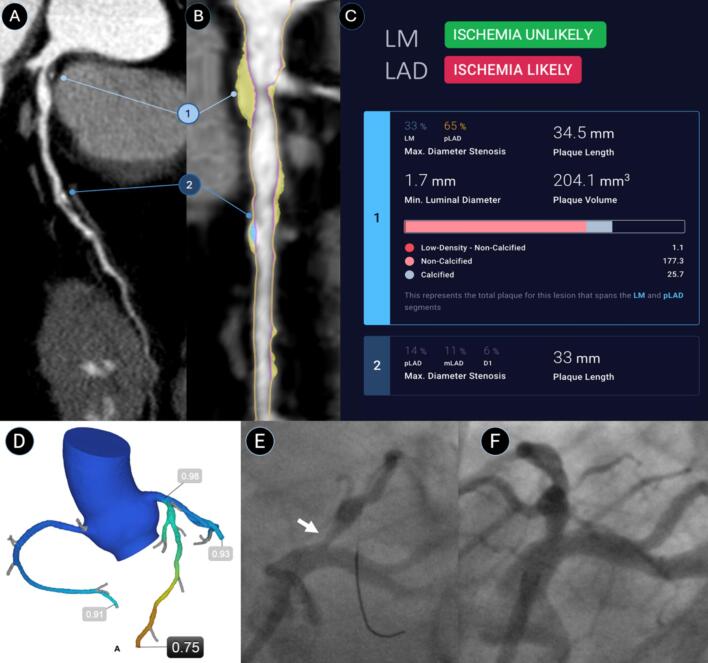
AI-QCT_ISCHEMIA_ case example. 50-year old male with new-onset stable chest pain. CCTA curved reformat depicts plaque in the LM and LAD (A). AI-QCT depicts predominantly non-calcified plaque (yellow overlay) in the LM/pLAD and mixed plaque (blue overlay) in the mid vessel (B). AI-QCT_ISCHEMIA_ depicts 2 plaques, one extending from the LM (33% stenosis) to the proximal LAD (65% stenosis), the second plaque has a maximal stenosis of 14% (C). AI-QCT_ISCHEMIA_ determined ischemia likely in the LAD, but not in the LM. This was concordant with a reduced FFR_CT_ of 0.75 (D). It was also concordant with invasive angiography where both a 70% stenosis as well as a reduced invasive FFR of 0.67 were found in the pLAD (E). The patient underwent successful placement of a drug-eluting stent with TIMI-3 flow result (F). AI-QCT, atherosclerosis imaging-quantitative coronary computed tomography; CCTA, coronary CT angiography; FFR, fractional flow reserve; FFR_CT_, fractional flow reserve from CT; LM, left main; LAD, left anterior descending; TIMI, Thrombolysis in Myocardial Infarction. (For interpretation of the references to colour in this figure legend, the reader is referred to the web version of this article.)
